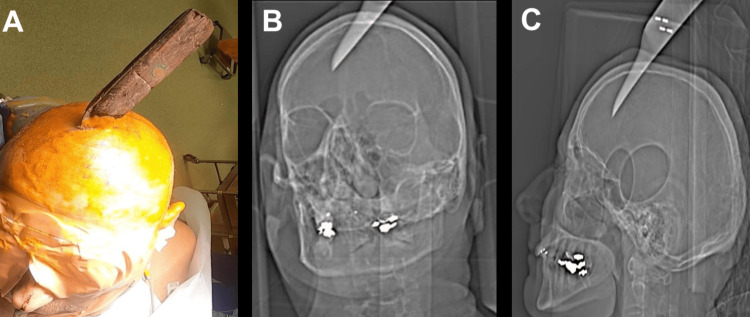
Preoperative photograph of case 1 in the operating room (A). Topograph skull X-rays of case 1 depicting bladed article penetrating the skull (B) and crossing the midline (C)
